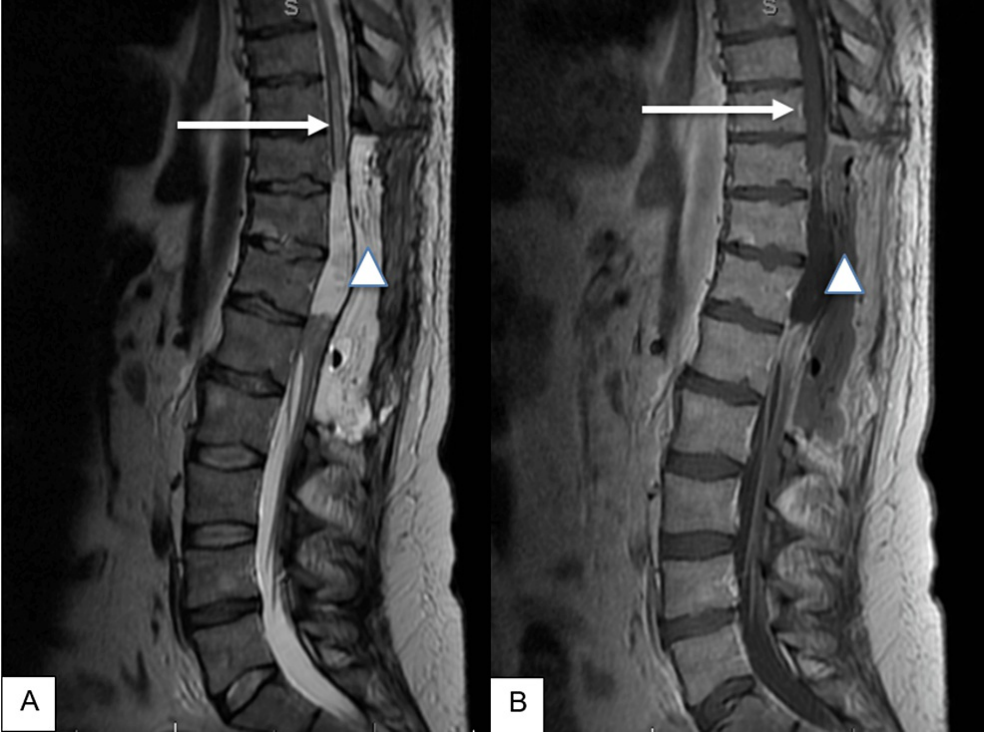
Post-operative MRI of the lumbar spine, sagittal view (A) T2-weighted and (B) T1-weighted post-contrast images showing a syrinx from the inferior border of T8 to mid-T10 (arrows) with a seroma (triangles) from the inferior border of T9 to L2 level.
